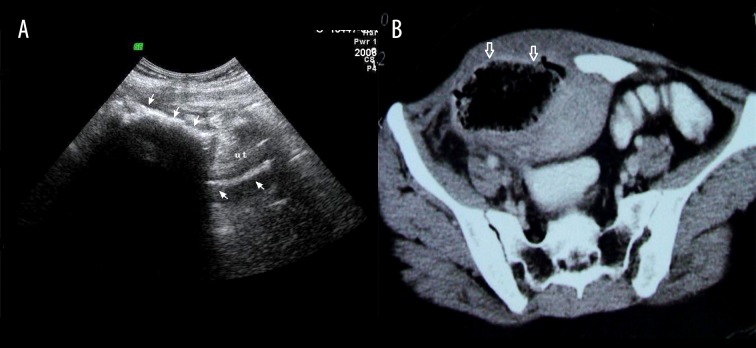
(A) Sonography shows a wavy, hyperechoic structure within the fundal region of the uterus. The endometrial echo of the lower uterine segment is well-delineated. (B) Contrast-enhanced CT at the level of the pelvis reveals a mass containing mottled, bubbly gas shadows protruding through the uterine fundus.
